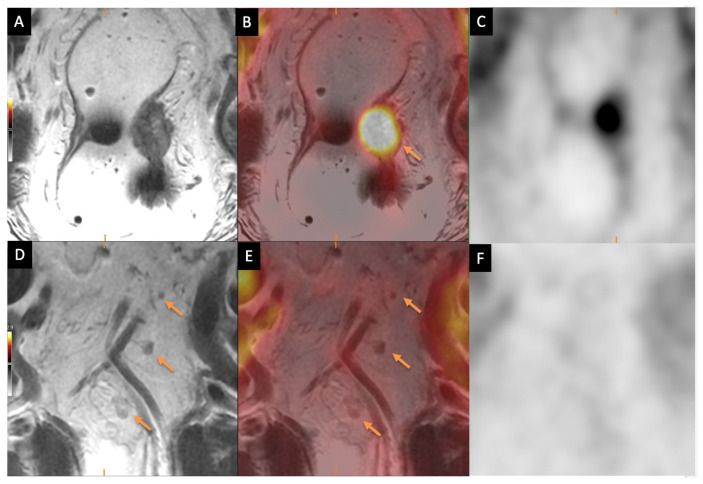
Rectal cancer staging with PET/MRI. (A) Initial MRI shows a T3 rectal adenocarcinoma centered at the high left rectal wall. (B) Fused [^18^F]FDG-PET/MRI images show focal FDG activity (arrow). (C) PET only images confirm focal activity in the rectal tumor. (D) MRI shows small nonspecific lymph nodes (arrows). (E) Fused [^18^F]FDG-PET/MRI images show no focal activity in lymph nodes, suggesting likely reactive and non-malignant nodes (arrows). (F) Corresponding PET-only images confirm no focal activity in lymph nodes.
